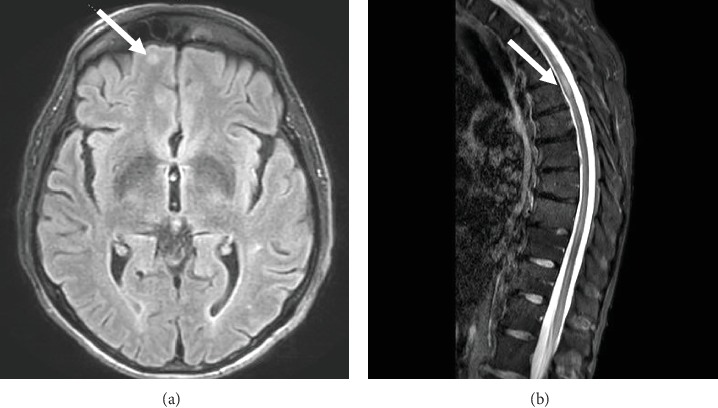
MRI with intravenous contrast of the brain and spine showing subacute infarcts in the bilateral cerebral hemispheres and left cerebellar hemisphere (a) and an enhancing lesion within the posterior aspect of the right hemicord at the T5 level (b).
